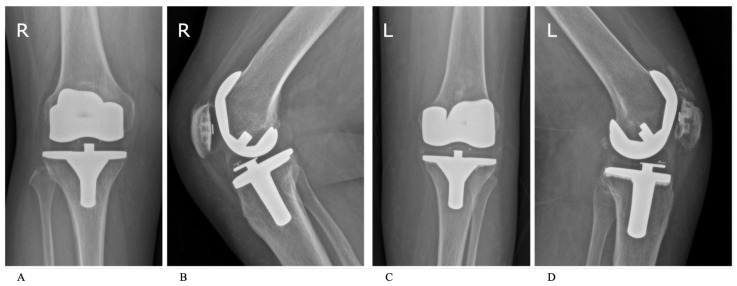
Anteroposterior and lateral radiographs of cementless (A,B) and hybrid cemented (C,D) TKA implants, 10 years postoperatively. “R” indicates the right knee; “L” indicates the left knee.
